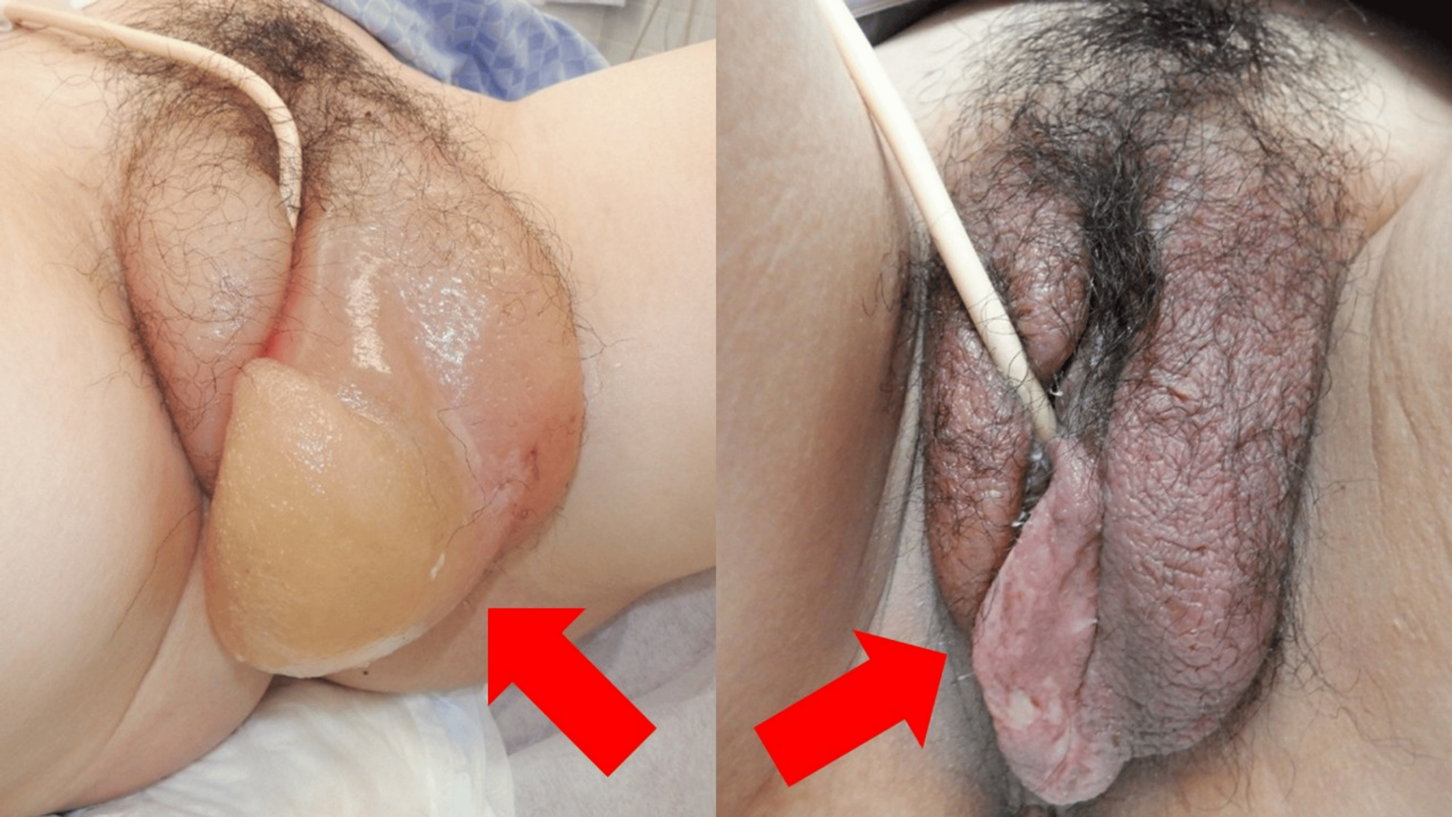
Clinical photographs of the vulva on admission (left) and on day 11 of hospitalization (right) Left image: Severe vulvar edema due to fluid extravasation, causing dysuria and impeding transvaginal ultrasound transducer insertion. Right image: Resolution of vulvar swelling following pregnancy termination and drainage of edema.
